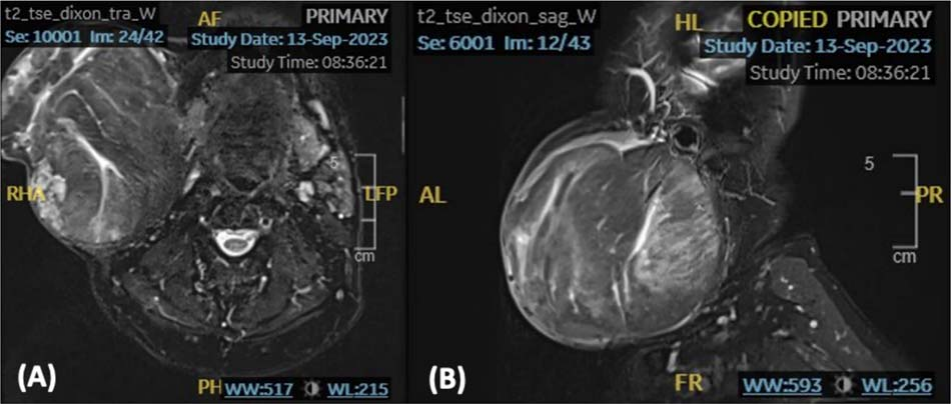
An axial (A) and sagittal (B) T2-weighted MRI showing a rounded well-defined heterogeneous lesion with soft tissue signal.
